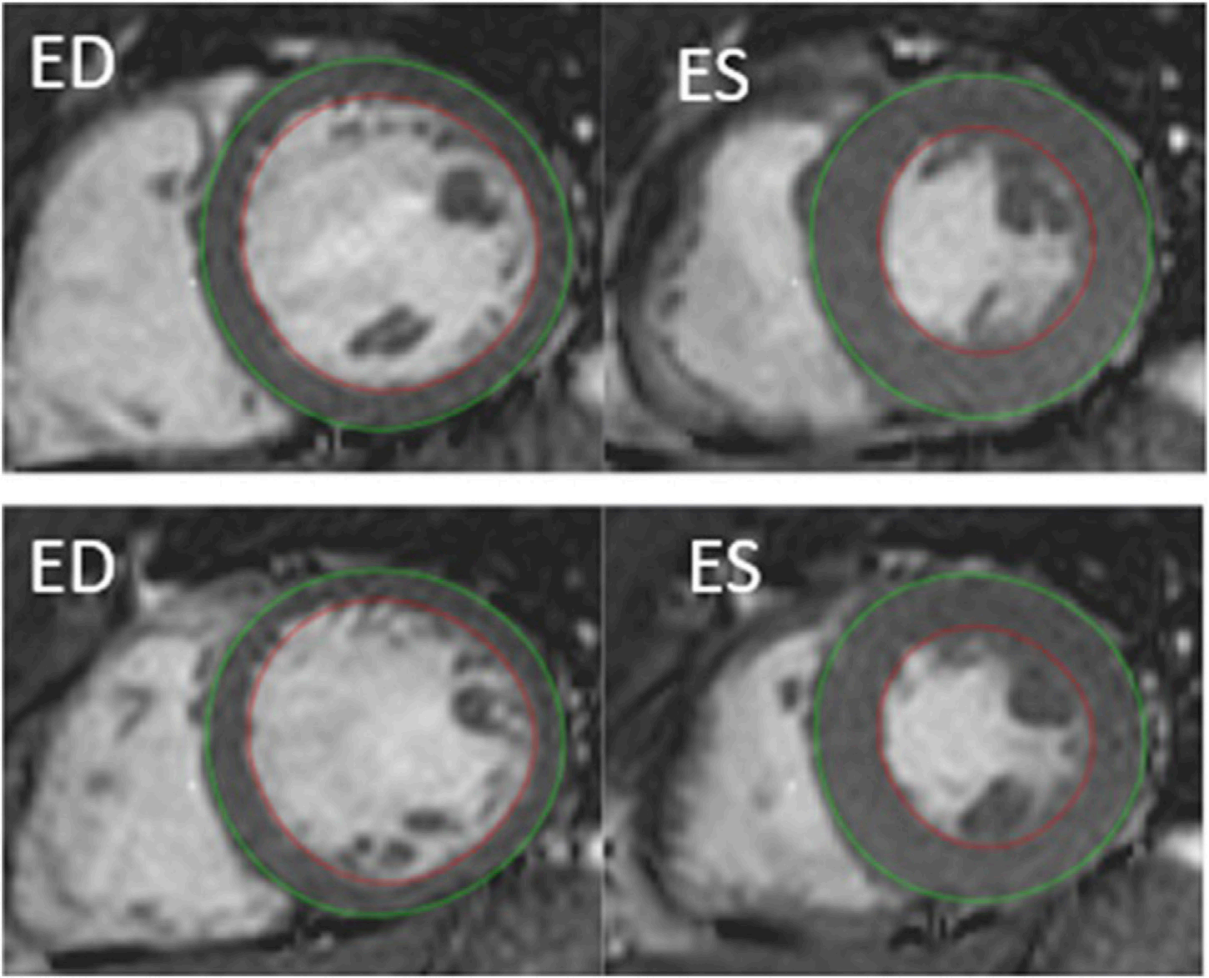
Example of cardiac MRI determined myocardial mass by semi-automatic segmentation of the left ventricle, short-axis view. Delineation of endocardial contour in red and epicardial contour in green. ED, end diastolic. ES, end systolic. Top: rest. Bottom: after 4 min of apnea.
